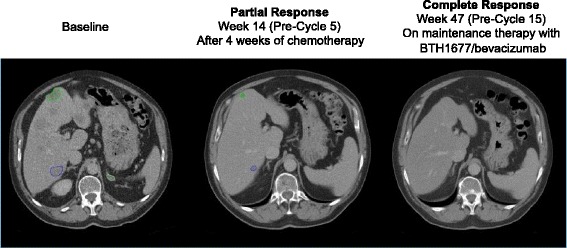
CT scans showing continued reduction in tumor burden while on BTH1677 maintenance therapy. Target lesion locations at baseline included left hilum, mediastinal lymph nodes, adrenals, and liver. The patient remained on study and in CR at the time of the primary analysis (19 weeks later). *Abbreviations: CR* complete response, *CT* computed tomography
